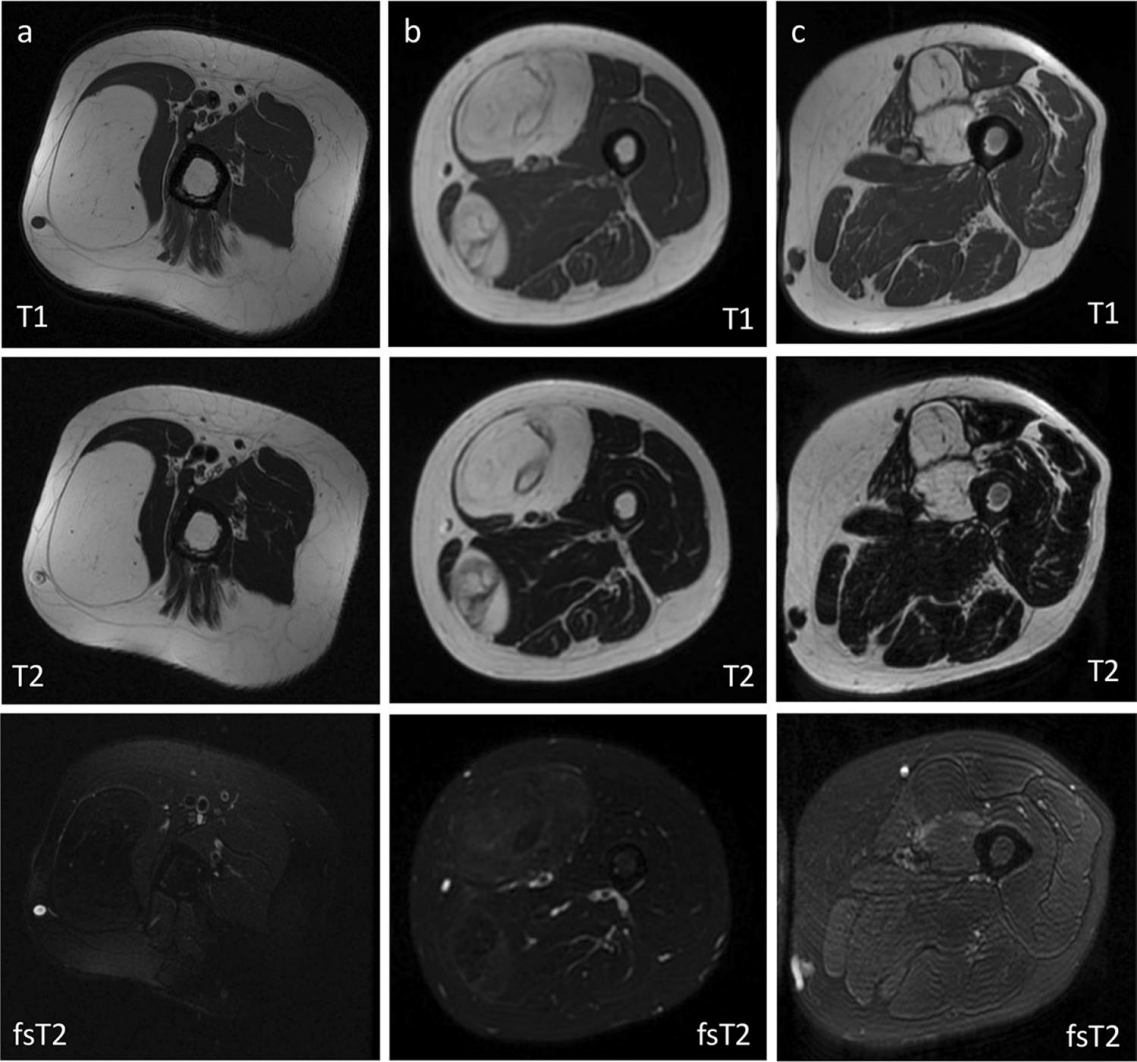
True negative (a, correctly classified lipoma), false positive (b, lipoma misdiagnosed as ALT) and true positive (c, correctly classified ALT) according to both radiomics-based machine learning and qualitative assessment performed by the radiologist. In (a), correctly classified lipoma shows homogeneous signal with complete fat suppression. Intralesional septations are seen in correctly classified ALT (c) but also lipoma misdiagnosed as ALT (b). Fat-suppressed T2-weighted sequences were used only for qualitative assessment performed by the radiologist. Radiomics-based machine learning analysis included T1- and T2-weighted sequences without fat suppression
